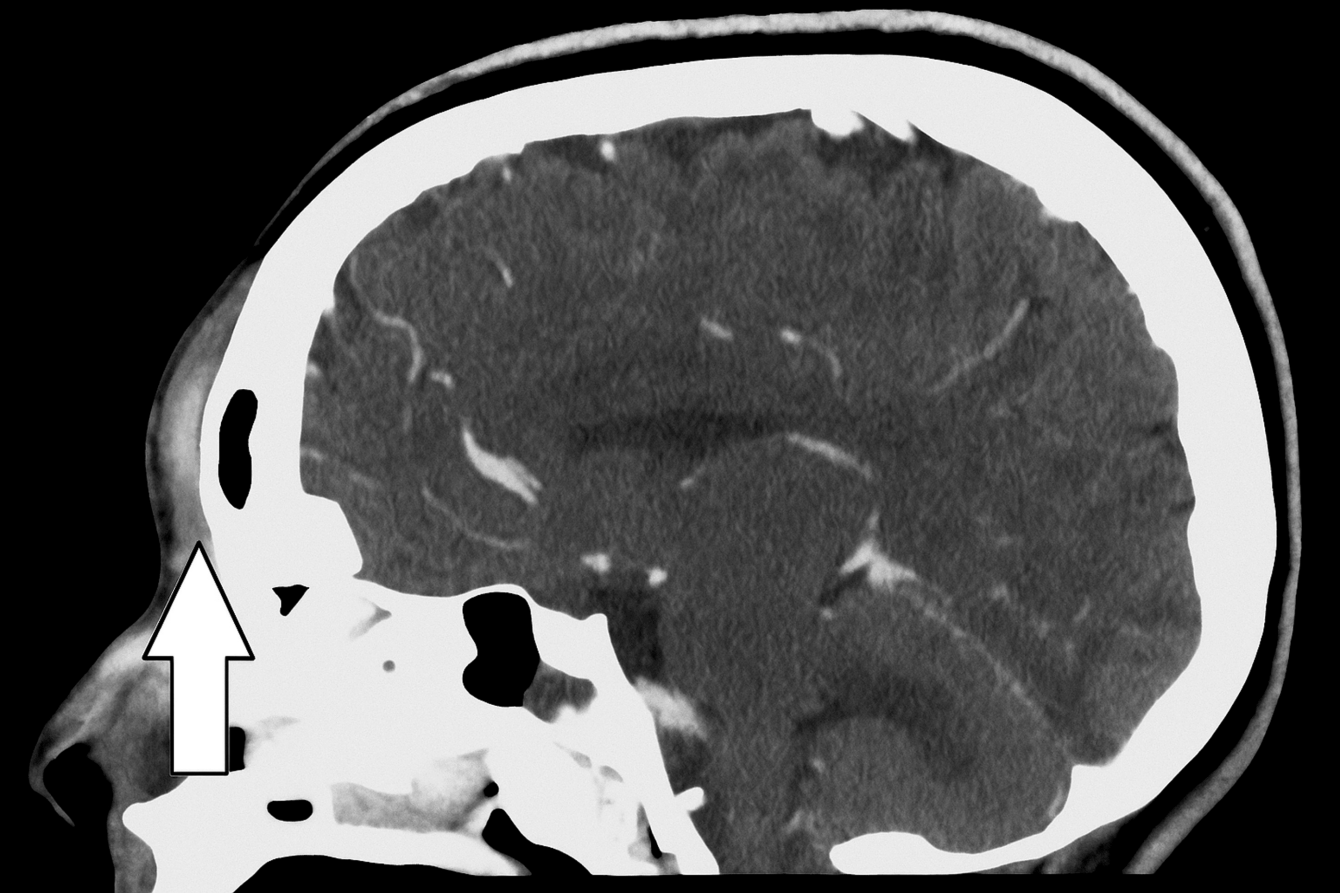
Head CT with contrast showing enhancing soft tissue thickening with a small abscess and air pocket (white arrow) over the anterior frontal region of the skull.
